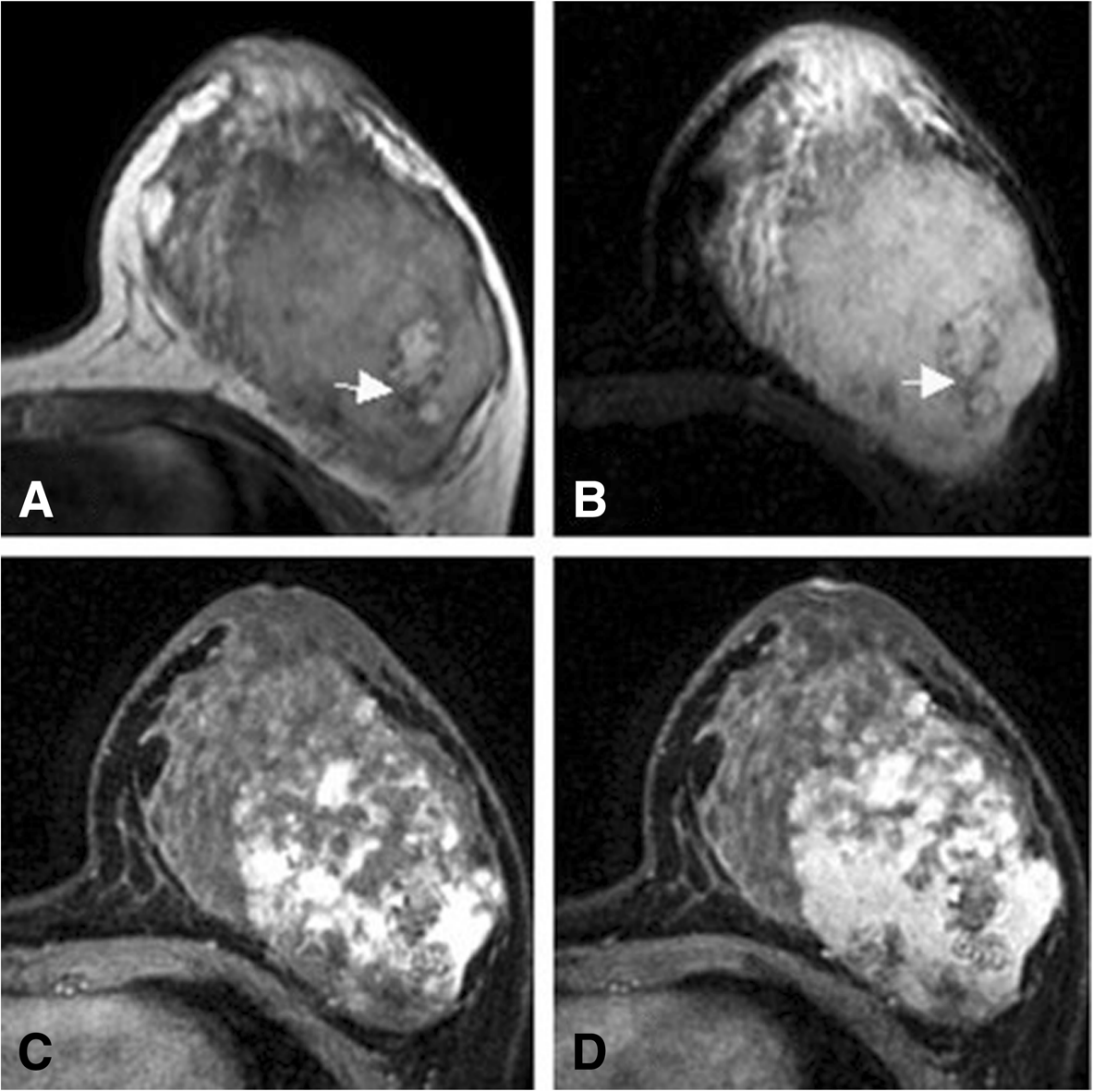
A female who had a mass in her left breast for 16 months. The mass has been enlarging in a high rate for three months since she was pregnant recently. The hemosiderin ring at the edge of the hemorrhagic lesion has long T1 and short T2 signals (white arrow). a: On T1-weighted images, the lesion shows heterogeneous signal intensity. b: On fat-suppressed T2-weighted images, the lesion shows bright (high) signal intensity. c: The contrast enhanced images demonstrate heterogeneous enhancement in the early stages. d: Concentric enhancement is observed in the delayed phase
